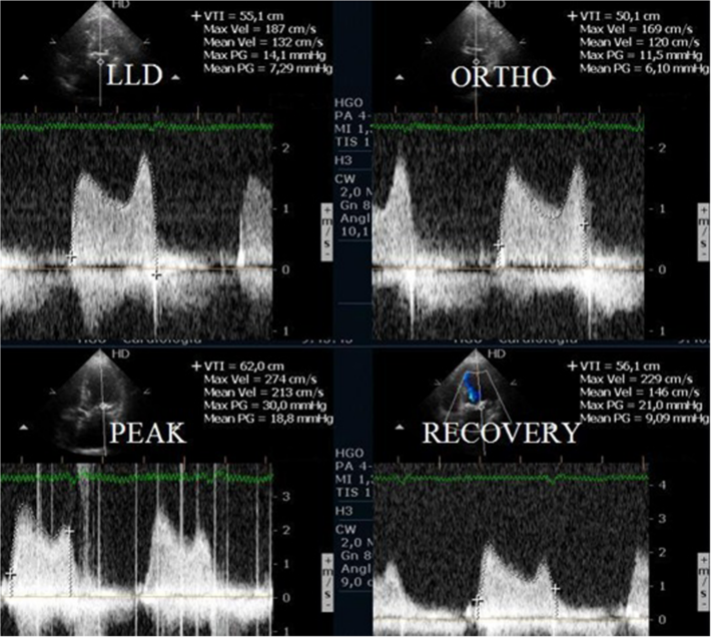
Left atrium/ left ventricle gradient, evaluated with CW Doppler, at different stages of the study in one patient with mitral stenosis.
